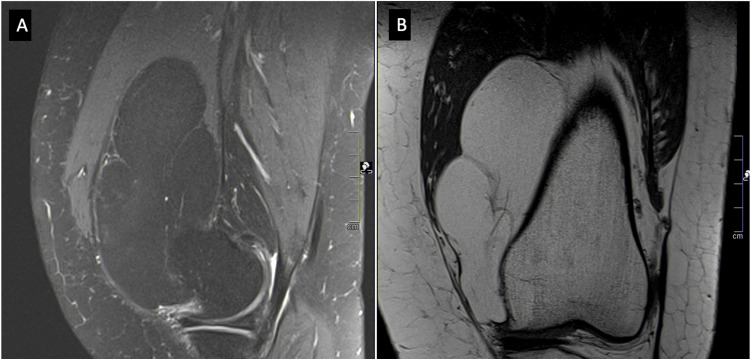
MRI images of the left knee (A) Sagittal PD FS MRI sequence (0.6 mm thickness) showing a hypointense signal, confirming the presence of intralesional fat. (B) Coronal T1-weighted MRI showing a homogeneous, well-defined hyperintense lesion, measuring 11 × 5 × 5 cm, with thin septations. PD, proton density; FS, fat suppression; MRI, magnetic resonance imaging
